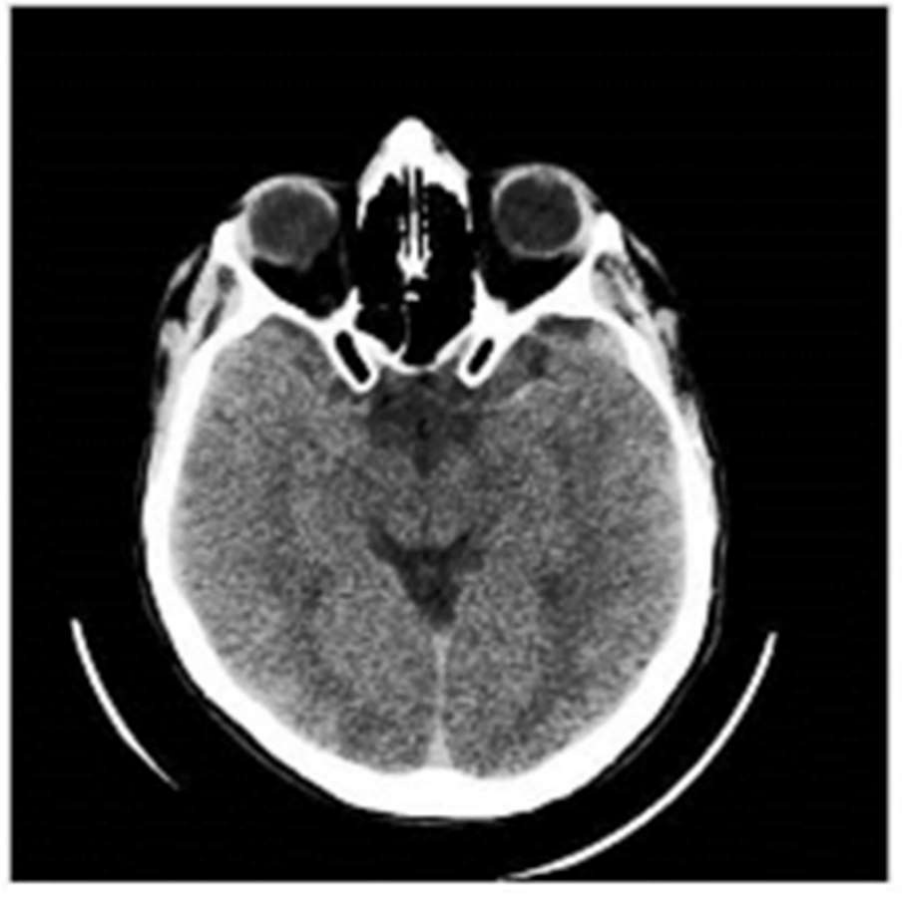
CT head showing a left hyperdense middle cerebral artery sign Computed tomography (CT) head with a left hyperdense middle cerebral artery sign
